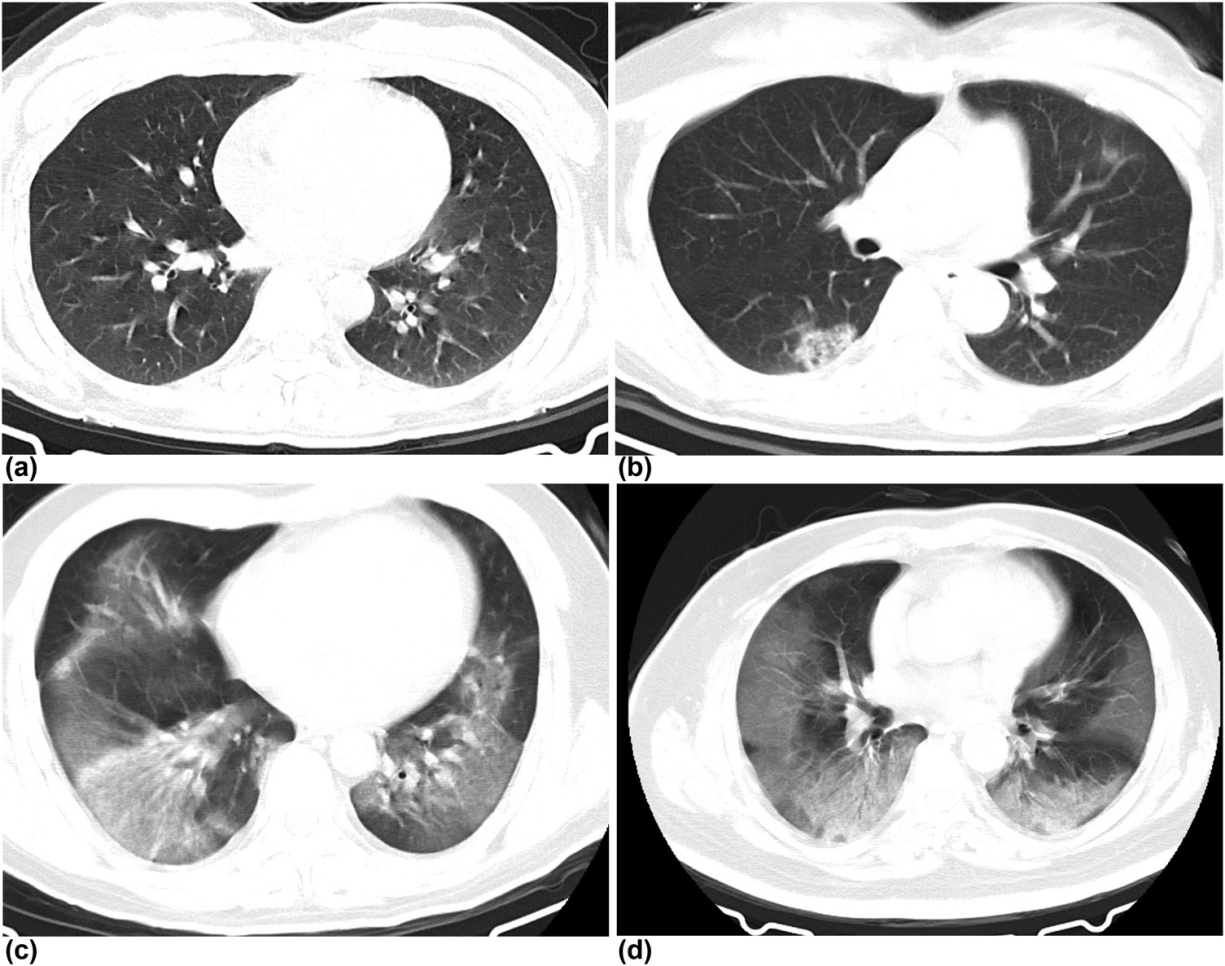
Chest CT images of four patients. (a) Transverse chest CT image from case 1 showing slight ground-glass opacity in the left lower lobe area on day 5 after symptom onset. (b) Transverse chest CT image from case 2 showing bilateral multiple patchy shadows with uneven density and unclear borders on day 7 after symptom onset. (c) Transverse chest CT image from case 3 showing bilateral multiple large diffuse ground-glass opacity on day 11 after symptom onset. (d) Transverse chest CT image from case 4 showing bilateral multiple large diffuse ground-glass opacity with obvious outer lateral distribution on day 11 after symptom onset.
